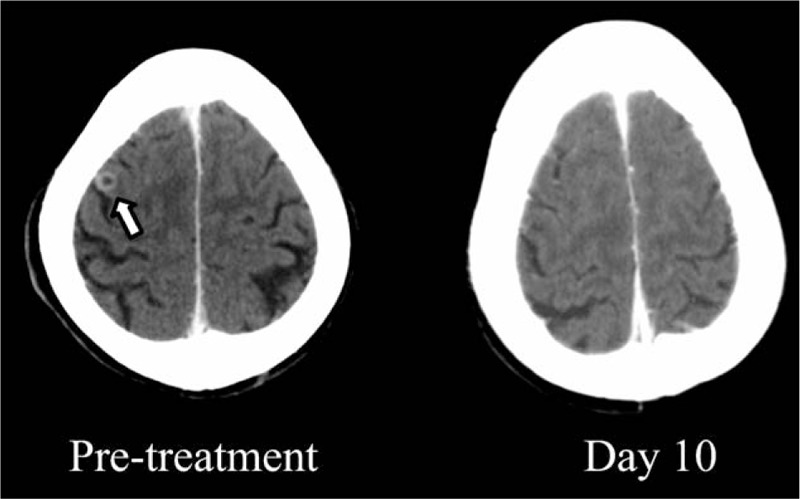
Contrast-enhanced axial brain CT images of case 2. Complete remission of a single brain metastasis (white allow) in the right parietal lobe after 10 days osimertinib administration.
